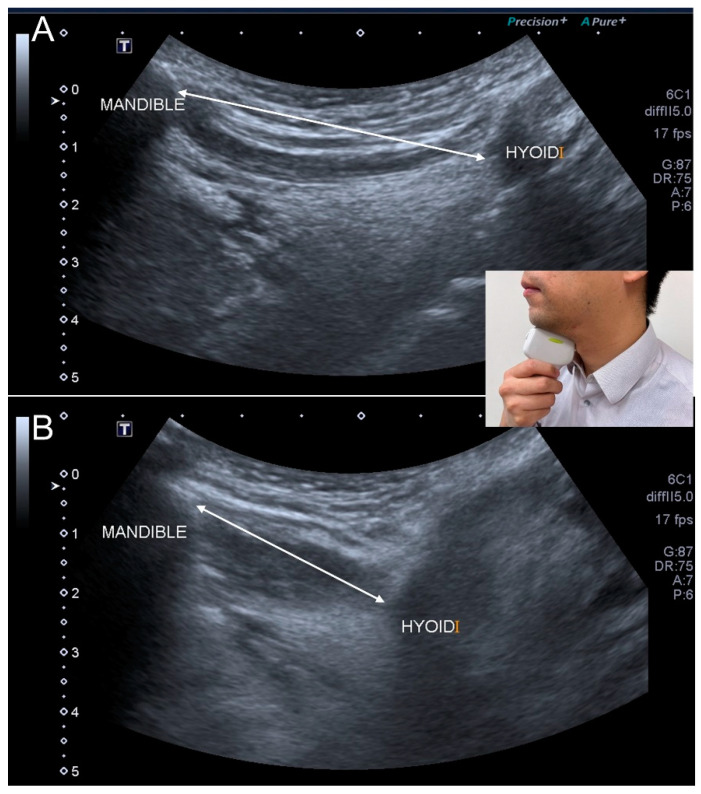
Ultrasonographic images show the hyoid movement at the onset of swallowing (A) and the moment of maximal displacement (B). Double-headed line, the distance between the mandible and hyoid bone.
